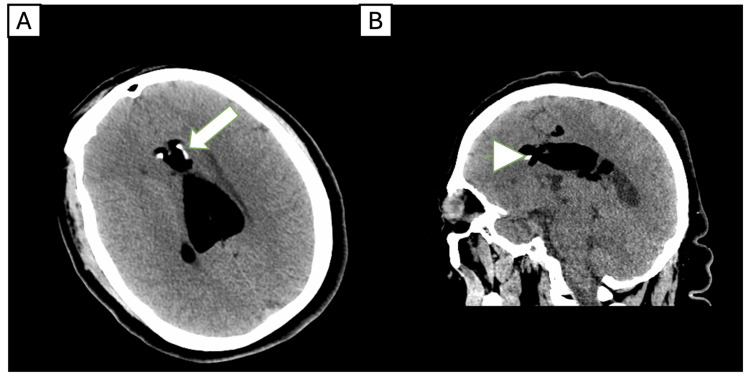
Non-contrast head CT was performed in 2024. Axial (A) and sagittal (B) images reveal a large, well-defined lipomatous lesion along the corpus callosum, demonstrating peripheral calcifications. The findings are consistent with a calcified pericallosal lipoma. CT: computed tomography
